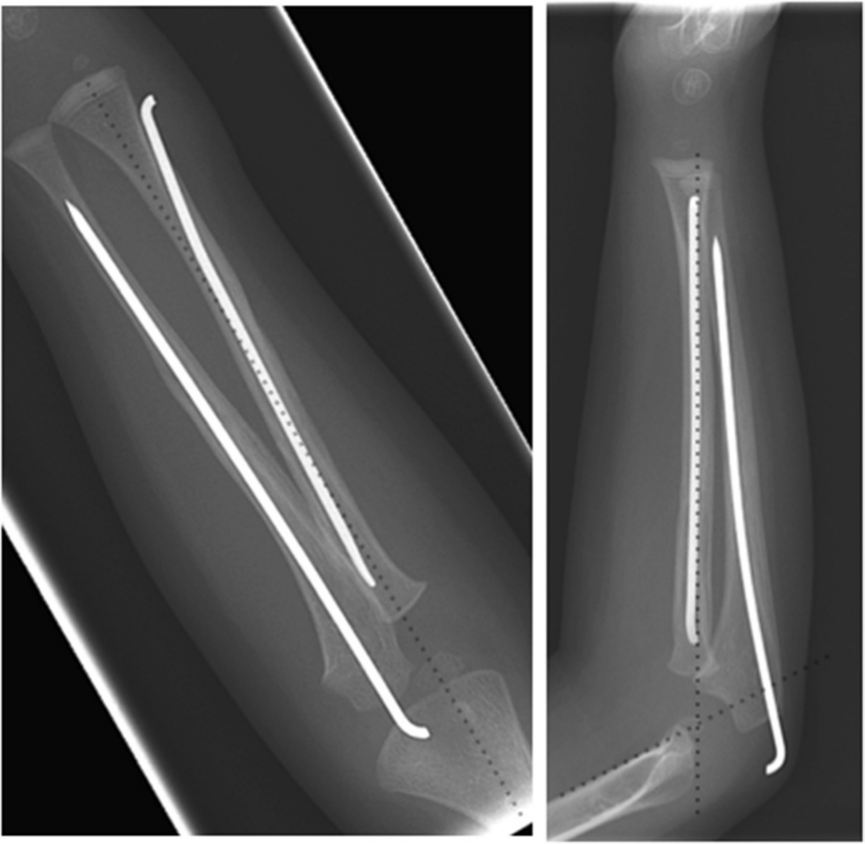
8 weeks post-operative radiographs demonstrating union of both radius and ulnar shaft fractures with successful maintenance of radiocapitellar joint reduction.
